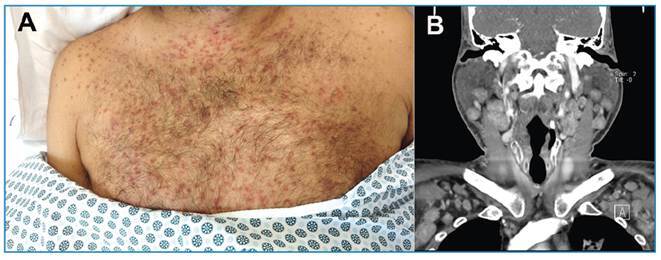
A. The upper trunk showing disseminated red and purple skin lesions. B. Computed tomography image of the cervical region showing bilateral and relatively symmetrical lymphadenopathy, affecting mainly the cervical and supraclavicular chains.
